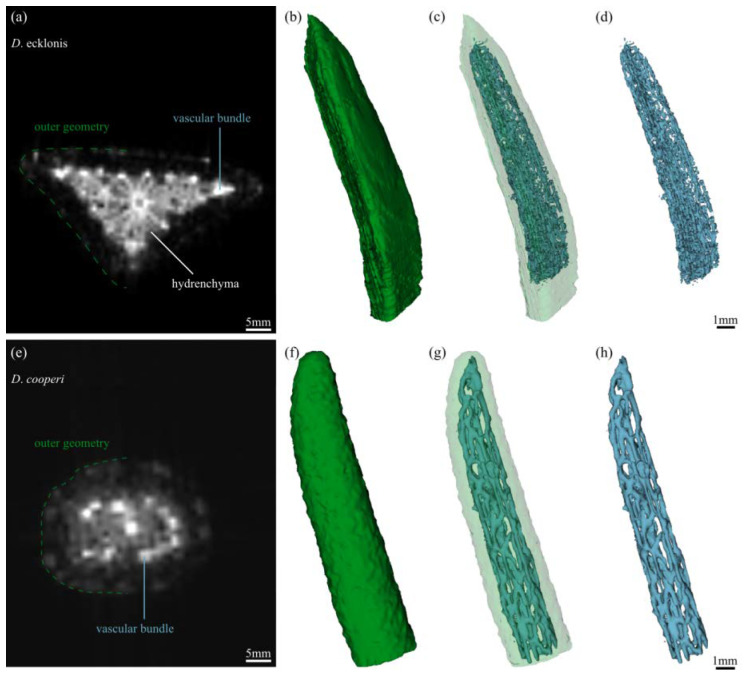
Magnetic resonance imaging of the leaf tips of *D. ecklonis* (a–d) and *D. cooperi* (e–h). (a) 2D tomogram (cross-section) of a *D. ecklonis* leaf. The contrasting agent (MnCl) does not provide sufficient contrast between large-celled hydrenchyma tissue and vascular bundles. (b–d) Quasi 3D data representation of the outer leaf geometry (b,c) and inner 3D vascular system (d) of the leaf of *D. ecklonis*. The low tissue contrast between the hydrenchyma and vascular bundles results in the reduced quality of the segmentation of the complex vascular network of the leaf in comparison with the results for *D. cooperi*. (e) 2D tomogram (cross-section) of a *D. cooperi* leaf. Bright appearance of vascular system by MnCl contrasting. (f–h) Quasi 3D data representation of the outer leaf geometry (f,g) and inner 3D vascular system (h) of the leaf of *D. cooperi*. The dotted line highlighting the outer geometry in (a,e) does not fully outline the entire leaf to allow better visualization of the image quality. Vascular anastomosis is frequent along the entire axis of the leaf within peripheral vascular bundles and between peripheral bundles and the central vascular strand.
